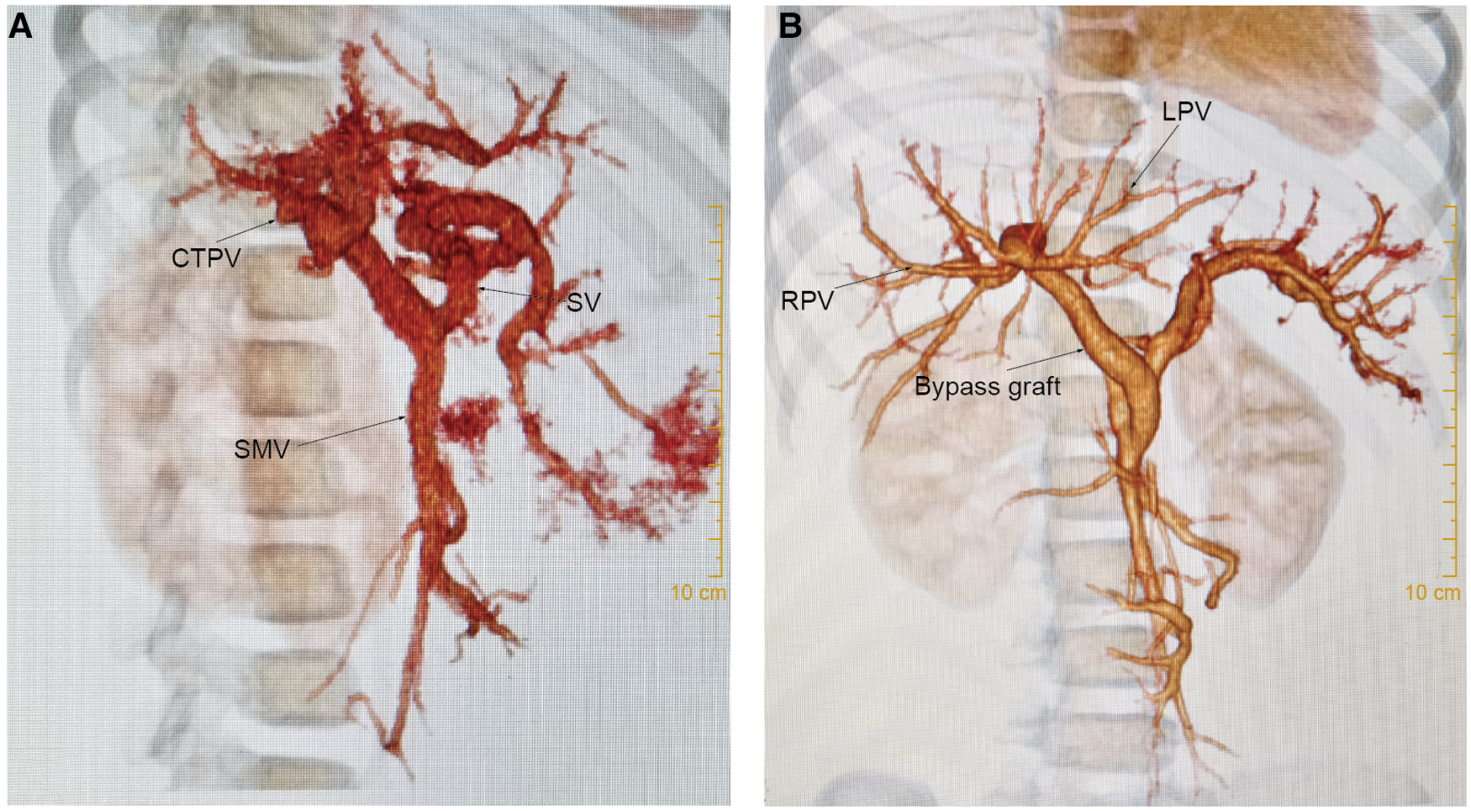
Ct scan. For case 8, (A): CT scan showed cavernous transformation of the portal vein before re-Rex shunt. (B): The bypass graft was patent 1 year after the re-Rex shunt.
